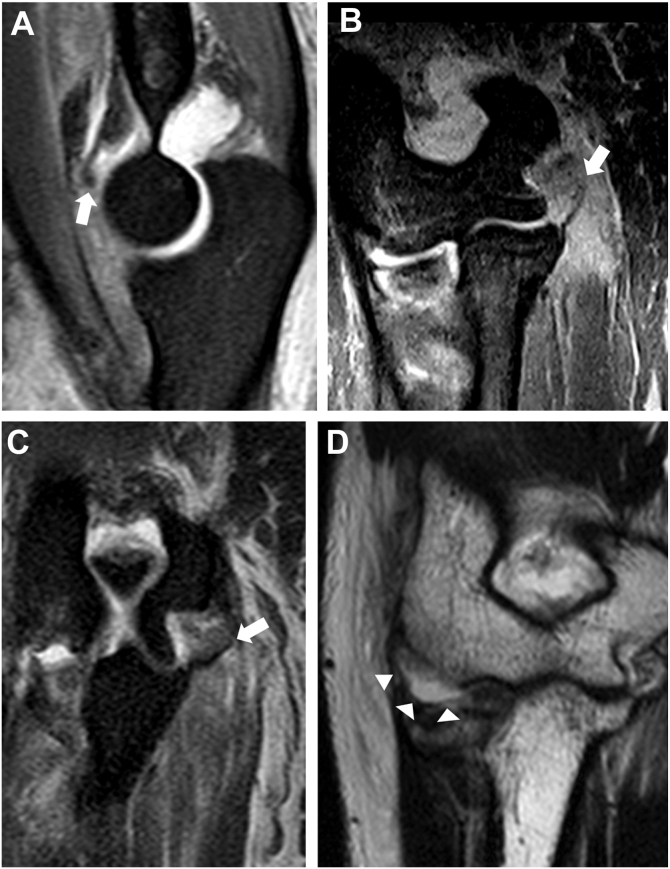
MRI findings of soft-tissue injuries. (A) Avulsion injury of the anterior capsule from the coronoid process. (B) Anterior medial collateral ligament tear from the medial epicondyle. (C) Detachment of the posterior medial collateral ligament from the posterior aspect of the medial epicondyle. (D) Lateral collateral ligament complex injury with distraction from the humeral origin. *MRI*, magnetic resonance imaging
